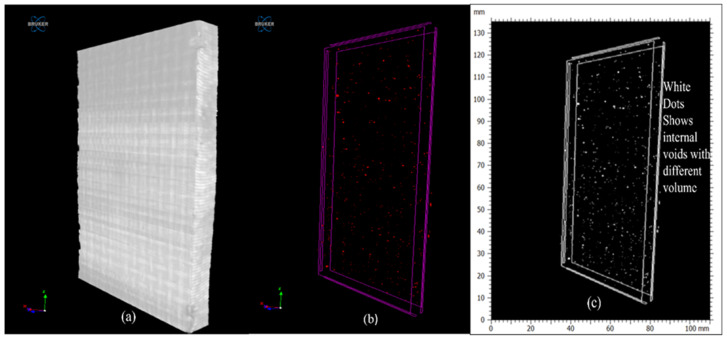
CT-scanned image of a ramie fiber panel with internal voids.
